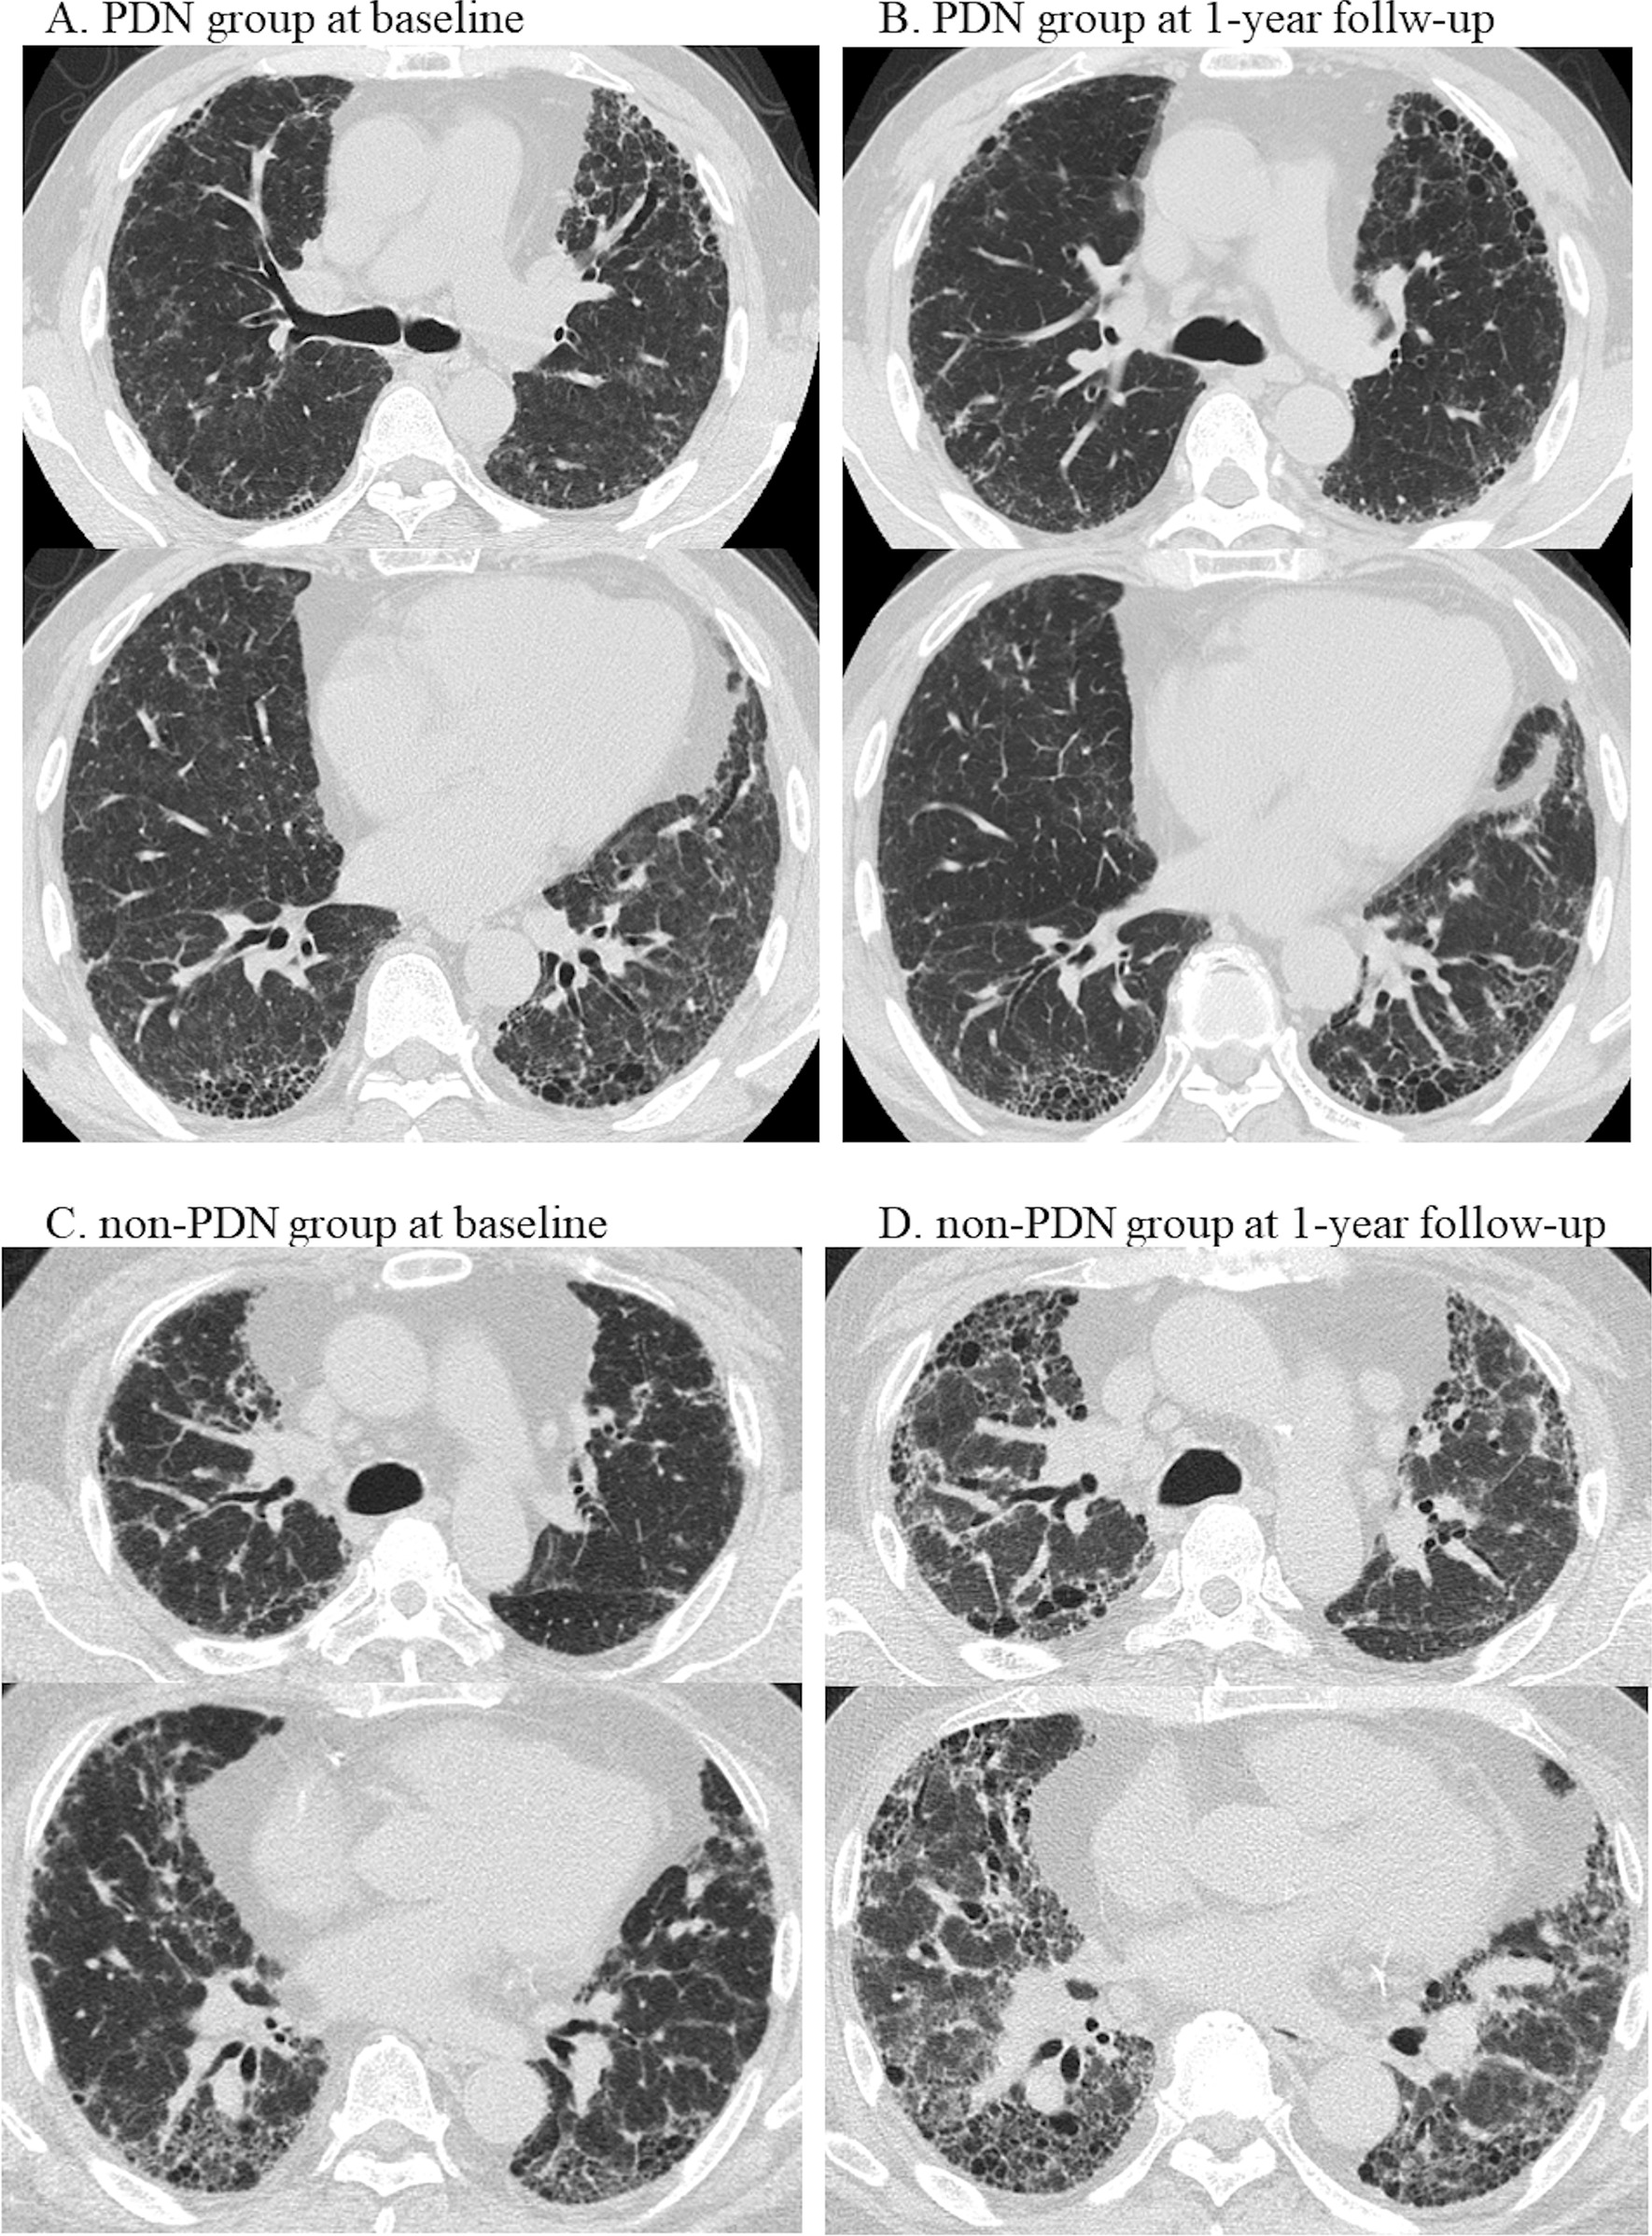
Representative matched pairs of patients. A, B A 63-year-old male with fibrotic bird-related hypersensitivity pneumonitis was treated with prednisolone from baseline until the end of the follow-up period at 60 months. At baseline, the patient’s %FVC was 57.9%, his annual %FVC decline before treatment was 26.1%, and his BAL lymphocyte count was 29%. At the 1-year follow-up, the change in %FVC from baseline was + 15.9%. A reduction in the extent of GGA with minimal fibrotic progression was observed on HRCT after one year. The total scores for the HRCT findings (baseline to the 1-year follow-up) were as follows: GGA (22 to 14), consolidation (2 to 0), reticulation (12 to 13), traction bronchiectasis (6 to 8), honeycombing (13 to 14), and mosaic attenuation (2 to 0). C, D A 66-year-old male with fibrotic bird-related hypersensitivity pneumonitis did not undergo treatment with corticosteroids and died at 17 months. At baseline, his %FVC was 46.6%, and his annual FVC decline until the date of diagnosis was 4.7%. At the 1-year follow-up, the change in %FVC from baseline was − 12.3%. Severe fibrotic progression was observed on HRCT. The total scores for the HRCT findings were as follows: GGA (11 to 18), consolidation (5 to 6), reticulation (16 to 19), traction bronchiectasis (10 to 15), honeycombing (14 to 19), and mosaic attenuation (0 to 0). *BAL* bronchoalveolar lavage; *%FVC* percent forced vital capacity; *GGA* ground glass attenuation; *HP* hypersensitivity pneumonitis; *HRCT* high-resolution computed tomography
